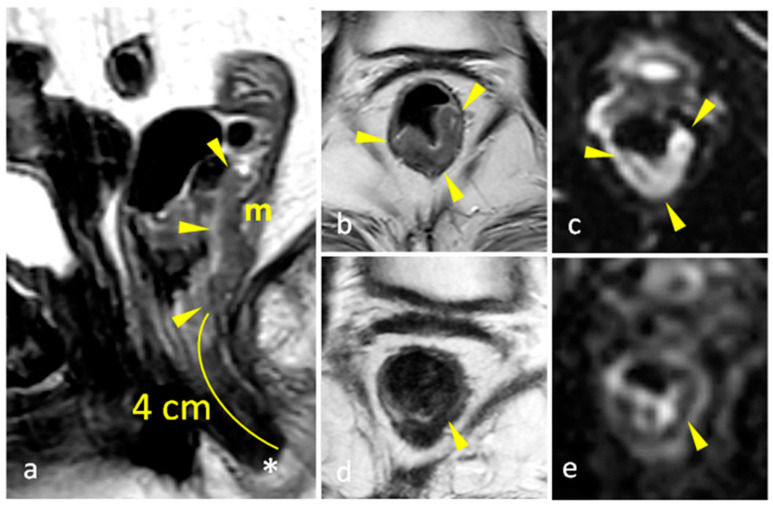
Pretreatment (a) sagittal T2-weighted TSE showing low rectal mass (m), seen 4 cm from the anal verge (*) extending for a craniocaudal length of 3.5 cm (between arrowheads) and showing intermediate signal intensity. (b) Axial T2-weighted TSE showing the rectal mass (arrowheads), involving the posterior and left lateral rectal walls, and extending from 1 till 8 o’clock in a clockwise direction. (c) Axial DWI of the same mass showing restricted diffusion. Post-treatment (d) axial T2-weighted TSE and (e) axial DWI at the same level, showing normal rectal wall and suggesting complete radiologic response (mrTRG 1).
